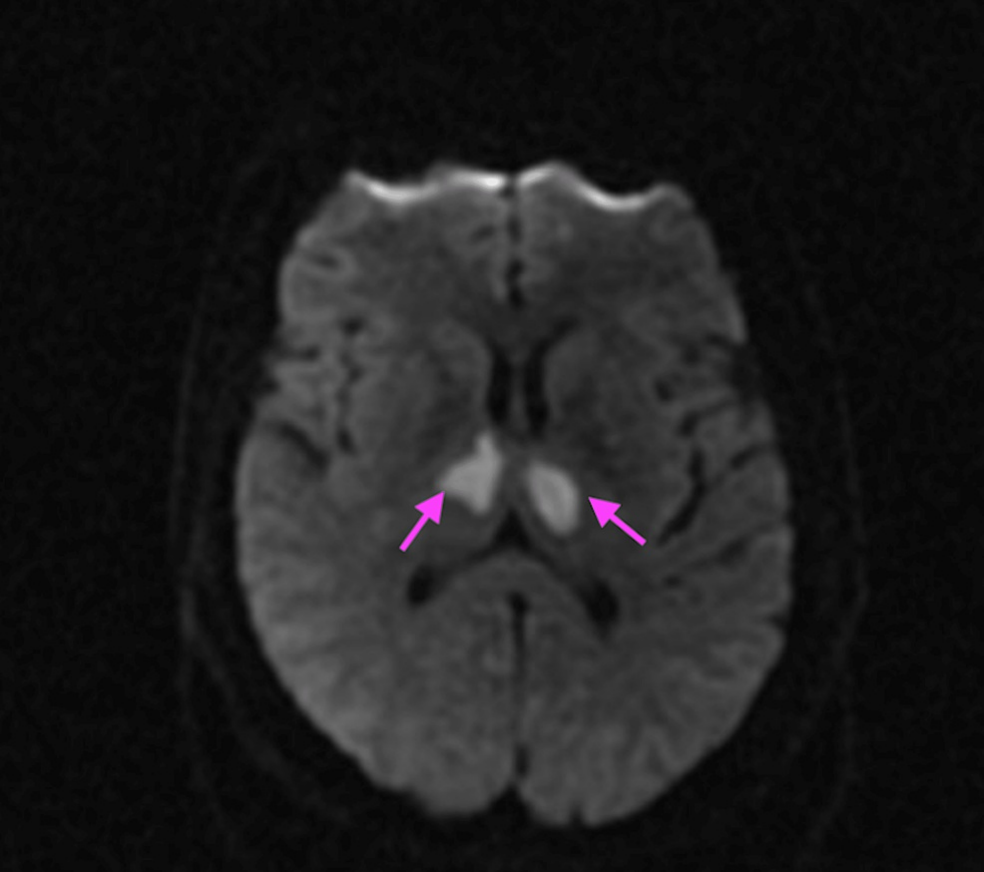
Bilateral thalamic infarcts were seen on magnetic resonance imaging (MRI) in Case 3
